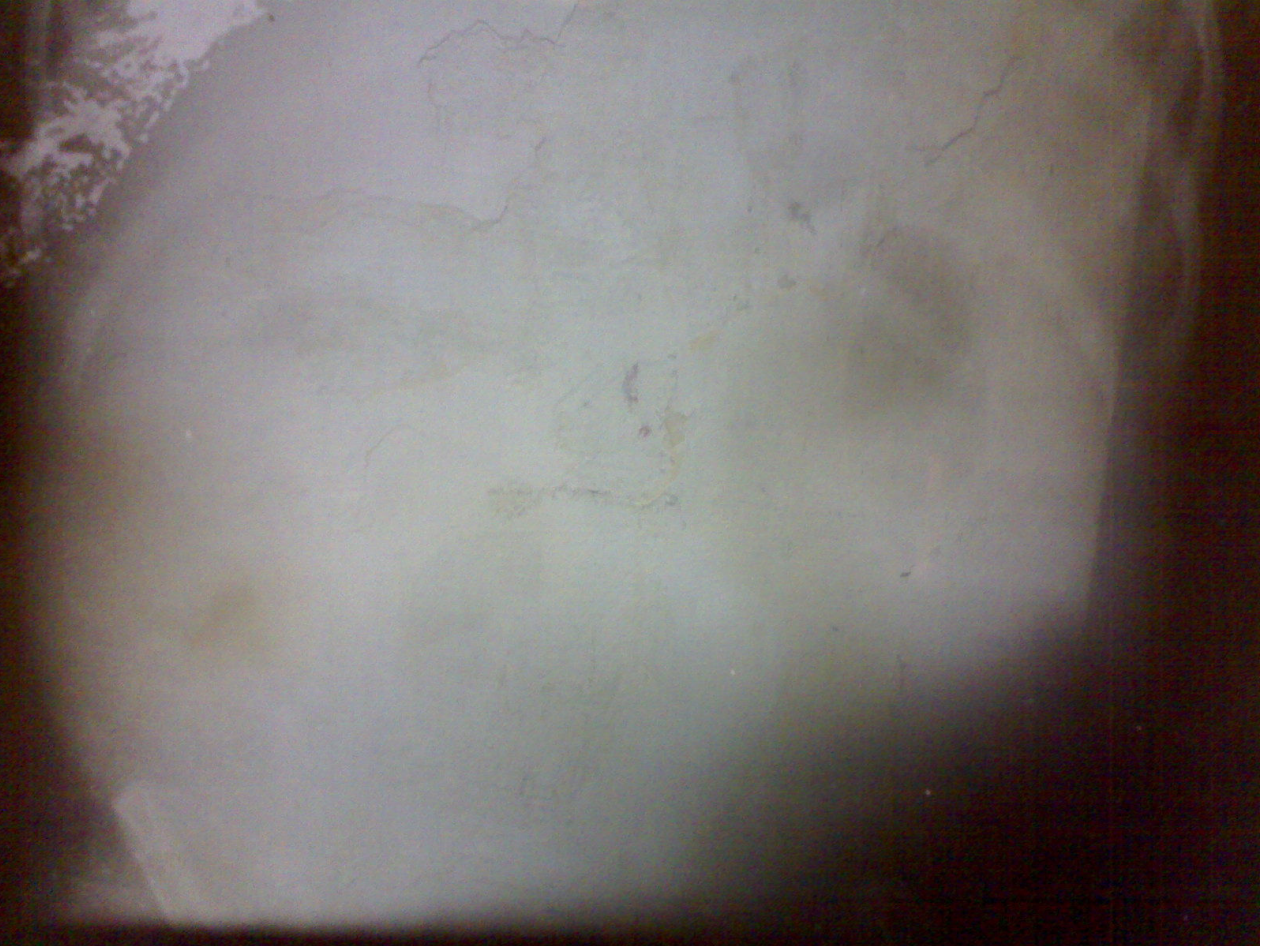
X-ray abdomen (erect) showing diffuse ground glass appearance.
